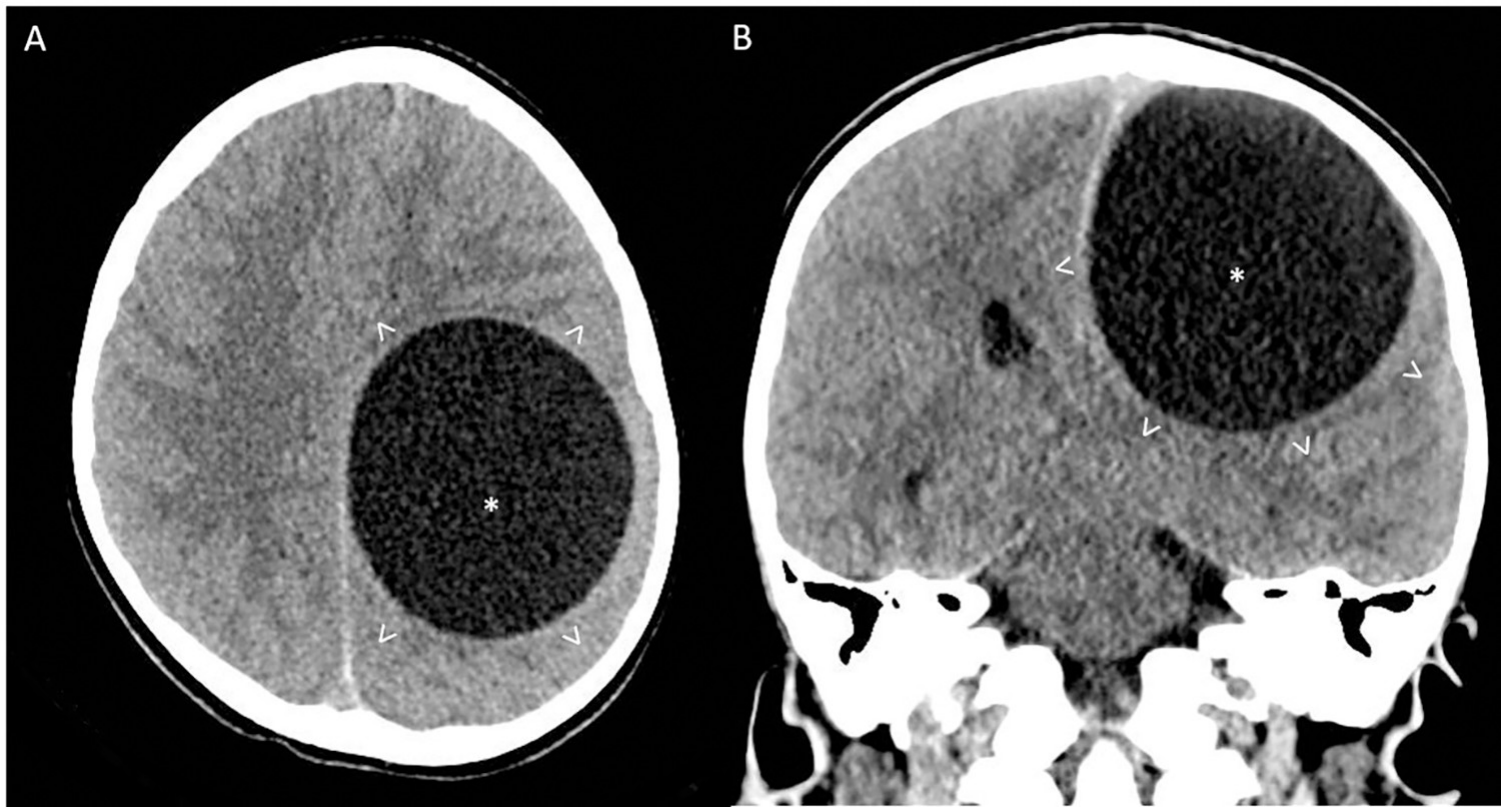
Computed tomography imaging without contrast obtained at presentation. Axial (A) and coronal (B) planes are shown. There is evidence of a round-shaped hypodense left frontal-parietal lesion (*) determining significant mass effect and no evidence of perilesional edema (arrowheads).
